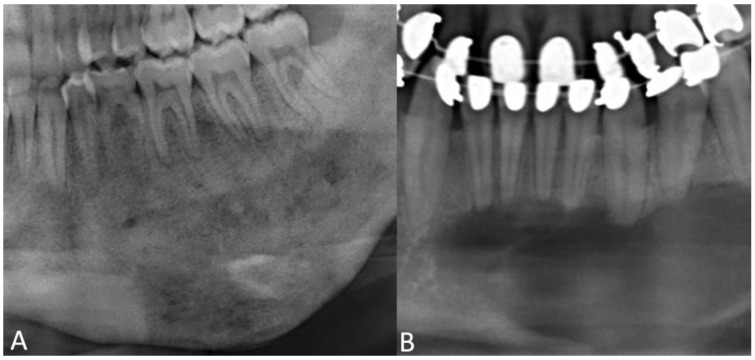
An excerpt of a panoramic radiography of a patient with chronic diffuse sclerosing osteomyelitis (A) and of a patient with central giant-cell granuloma of the mandible (B). (A) shows broad zones of sclerosing and subperiosteal bone formation. (B) shows well-defined and undulating borders.
